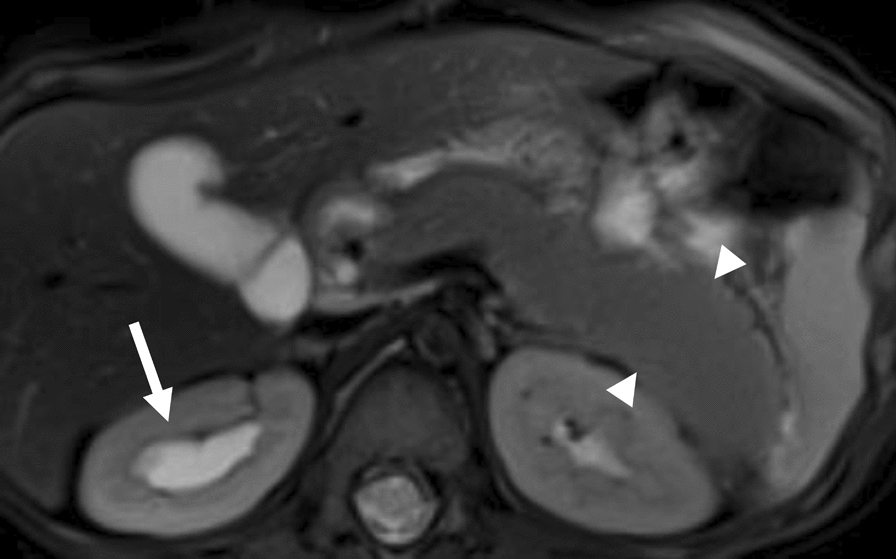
MRI of hydronephrosis and pancreatic hypertrophy in a 11-month-old boy with BWS^UPD^. The right renal hydronephrosis (arrow) and localized enlargement of the pancreatic body and tail (arrowhead) were observed on the fat-suppressed T2WI
